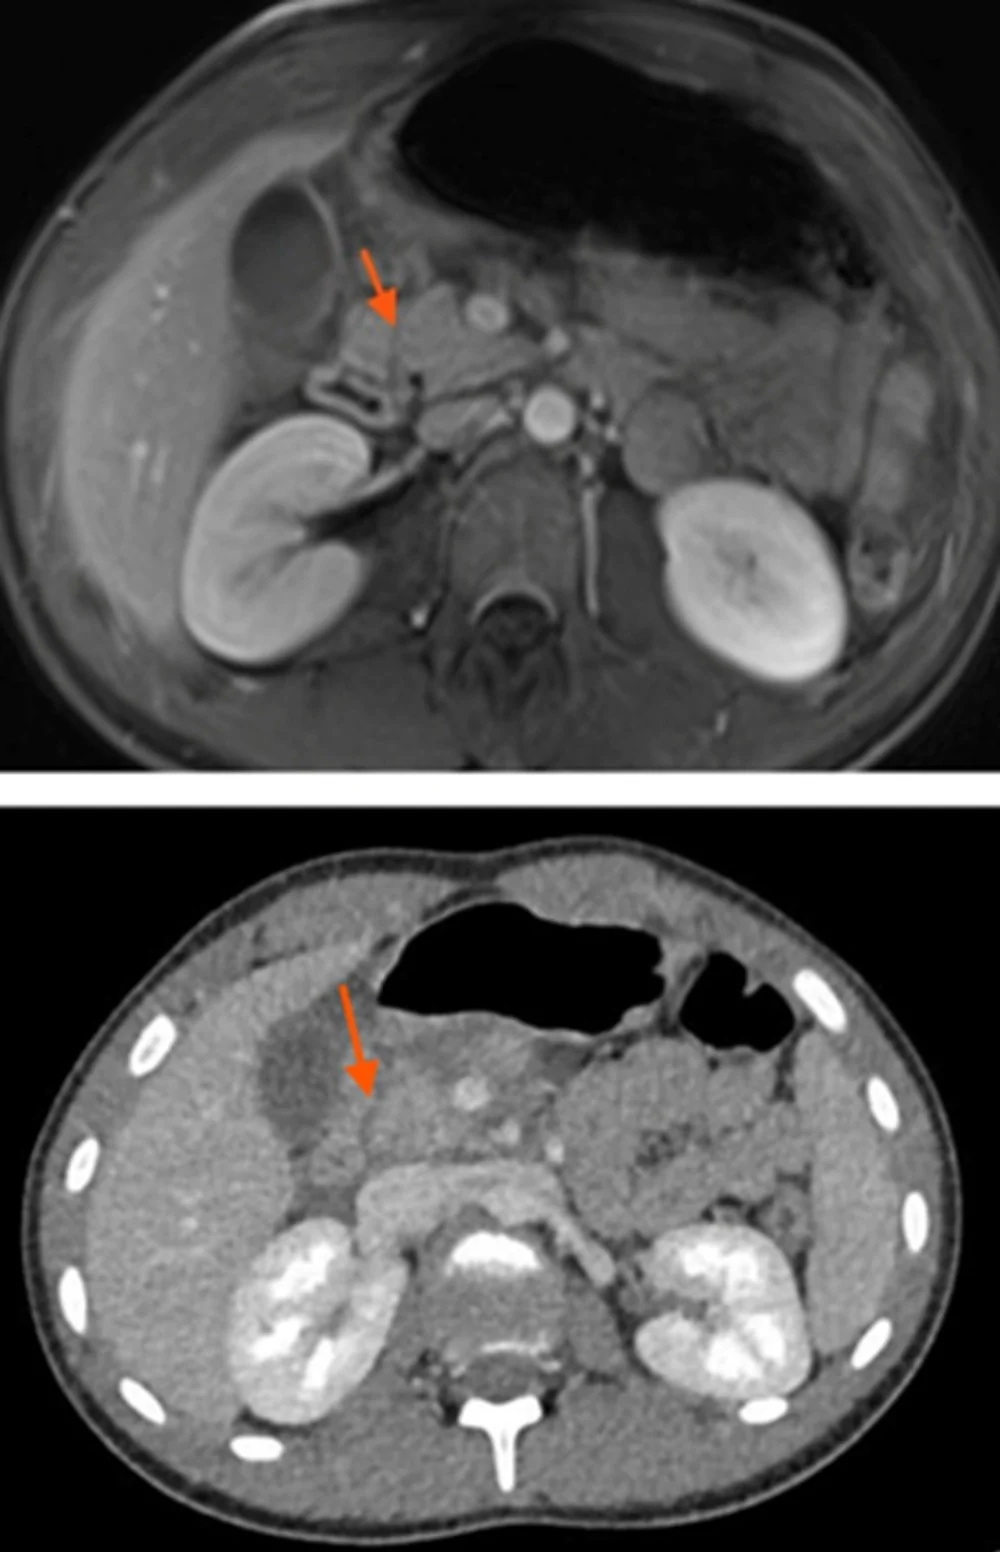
(Upper): MRI description of the complete transection of the neck of the pancreas, right of the mesenteric vessels. (Lower): Axial view of abdominal CT scan depicting Grade IV complete transection of the pancreas at the neck of the gland (Arrows indicate a pancreatic injury).
